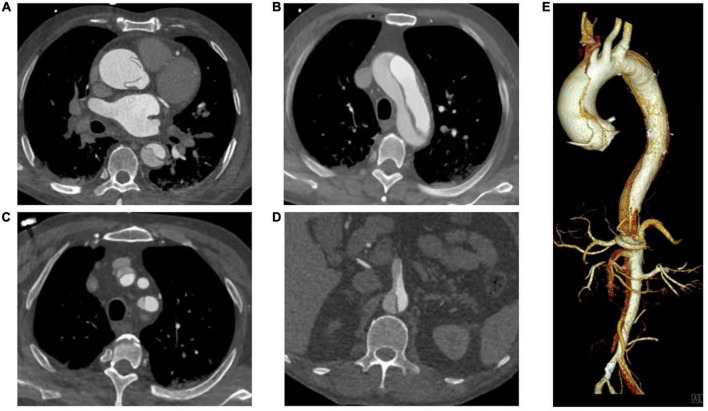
CT Angiography of the Aorta. (A) Ascending aorta; (B) Aortic arch; (C) Branch of aortic arch (involving brachiocephalic trunk and left subclavian artery); (D) Aortic dissection involving superior mesenteric artery; (E) Volumetric CT scanning: 3D reconstruction.
